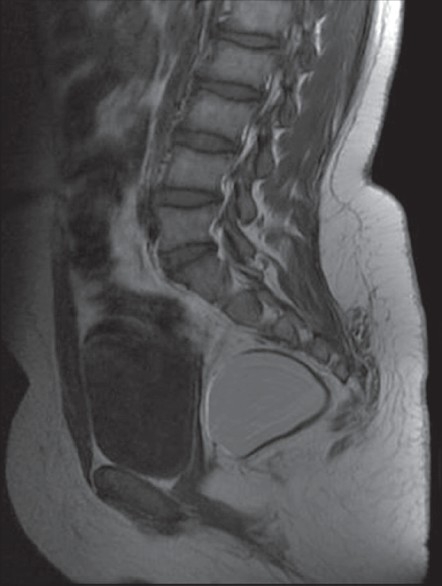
The pelvic T1-weighted magnetic resonance imaging in the sagittal slice showing a presacral mass with rectal compression
